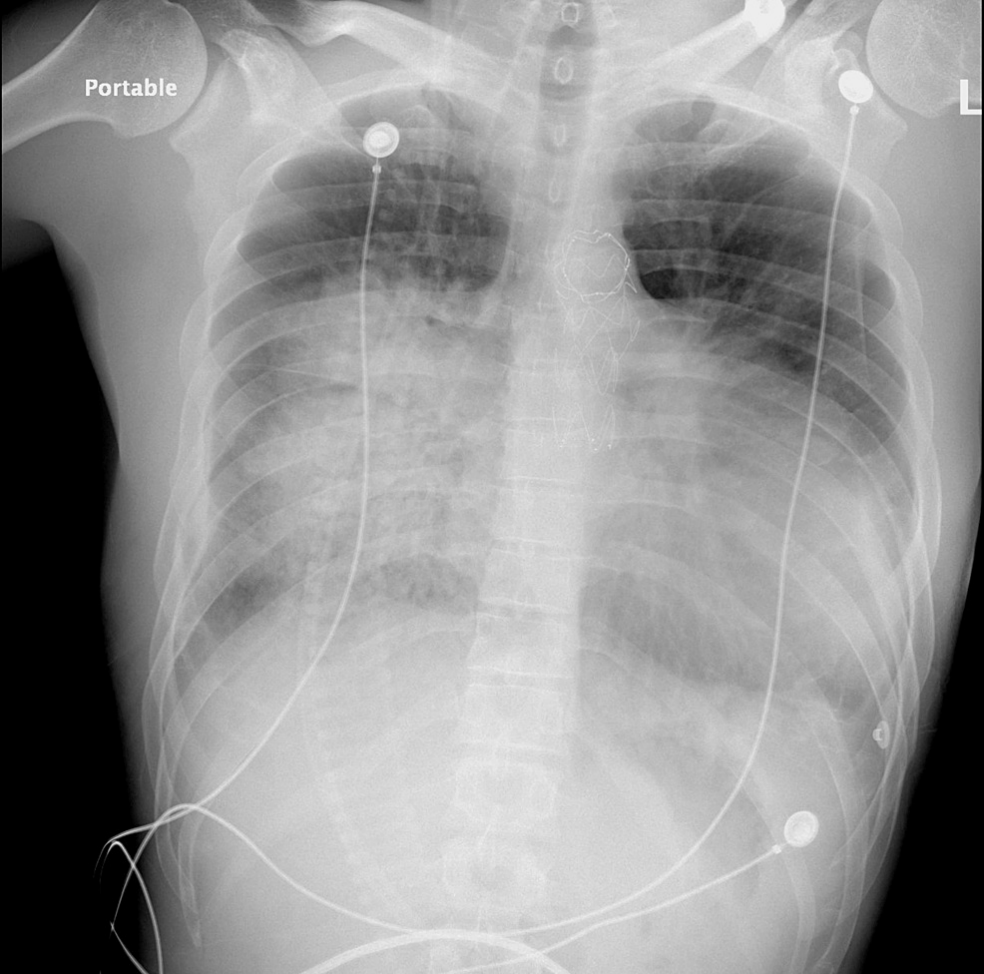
Chest X-ray AP view showing bilateral infiltrates and a left pleural effusion. AP, anteroposterior
